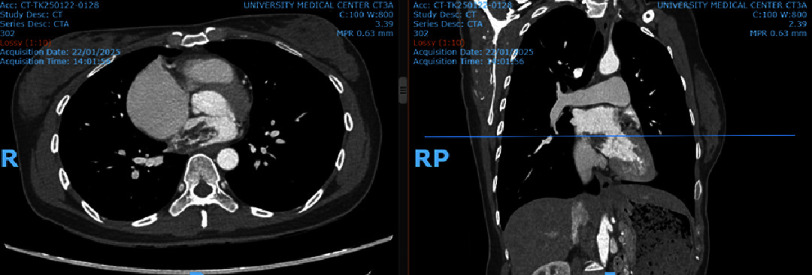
CT imaging demonstrated a left atrial myxoma measuring (20 ×30 mm), attached to the interatrial septum.
